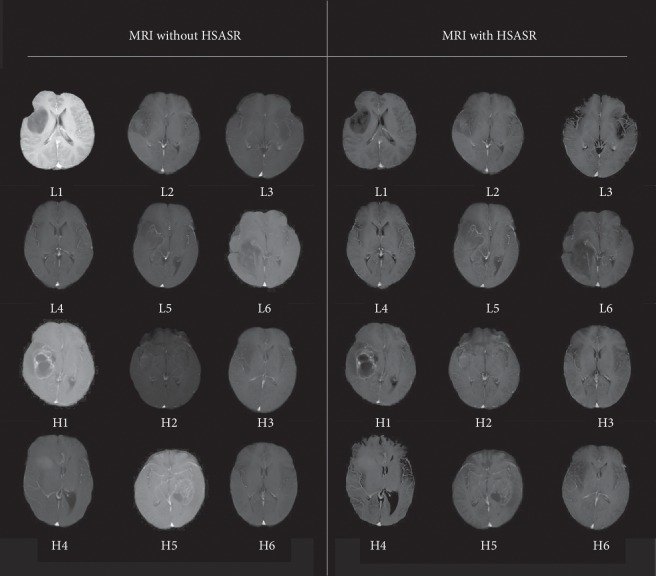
MRI comparison map before and after HSASR.
